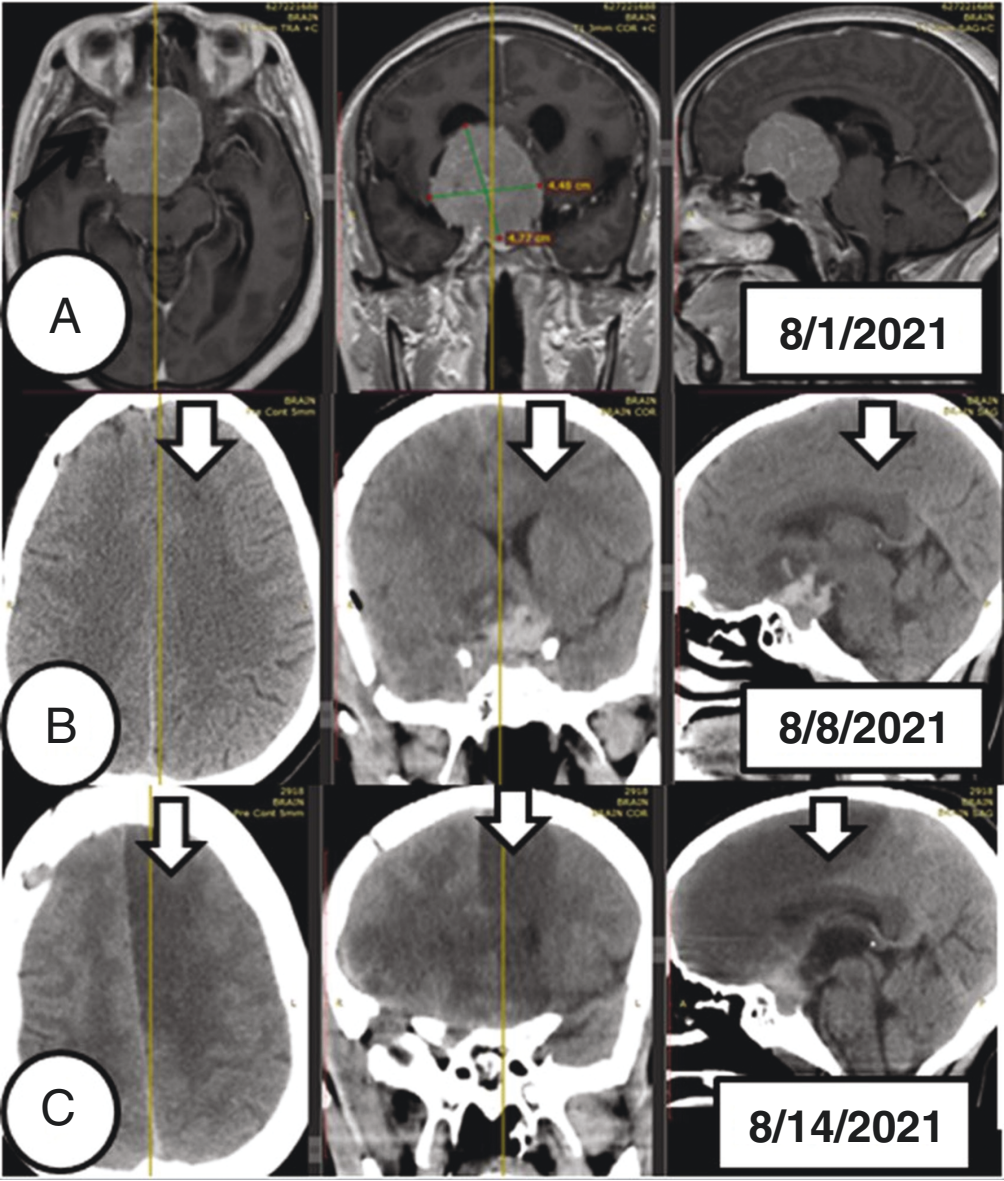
This image depicts the interval radiologic changes in a patient who was diagnosed to have delayed cerebral infarction due to both clinical and radiologic vasospasm after undergoing surgery for tuberculum sella meningioma. The image in (A) showed a giant-size tuberculum sella meningioma partly encasing the right middle cerebral artery. The first postoperative follow-up CT scan in (B) was obtained after 24 hours of the surgery and the down-pointing arrow confirms the absence of iatrogenic vascular injury. The CT in (C) was obtained after 6 days of the first follow-up CT as the patient presented with a new onset of seizure and right-side lower extremity weakness. The down-pointing arrow in (C) depicted left anterior cerebral artery territory infarction secondary to delayed vasospasm.
